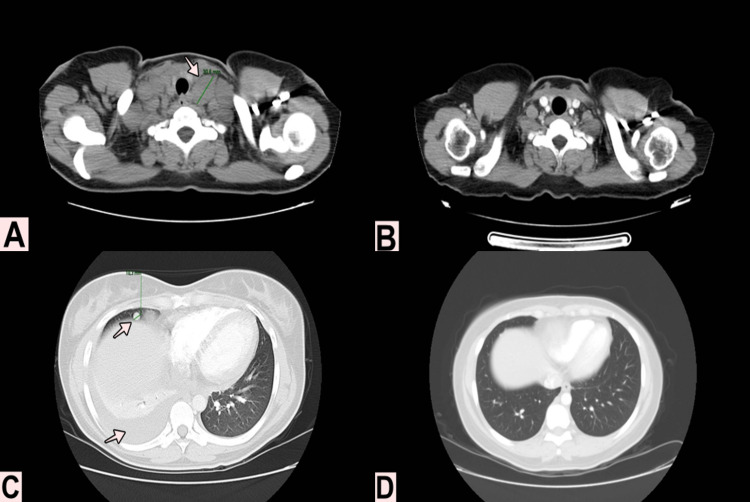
Imaging studies findings A. Neck CT from August 2018 showing a supraclavicular lymph node of 30mm. B. Neck CT from October 2019 with the resolution of supraclavicular lymph node involvement. C. Chest CT from August 2018 where right pleural effusion and pulmonary involvement were observed with a solid nodule of 10mm located in the right middle lobe. D. Chest CT from October 2019 with the resolution of the lung involvement and the pleural effusion.
